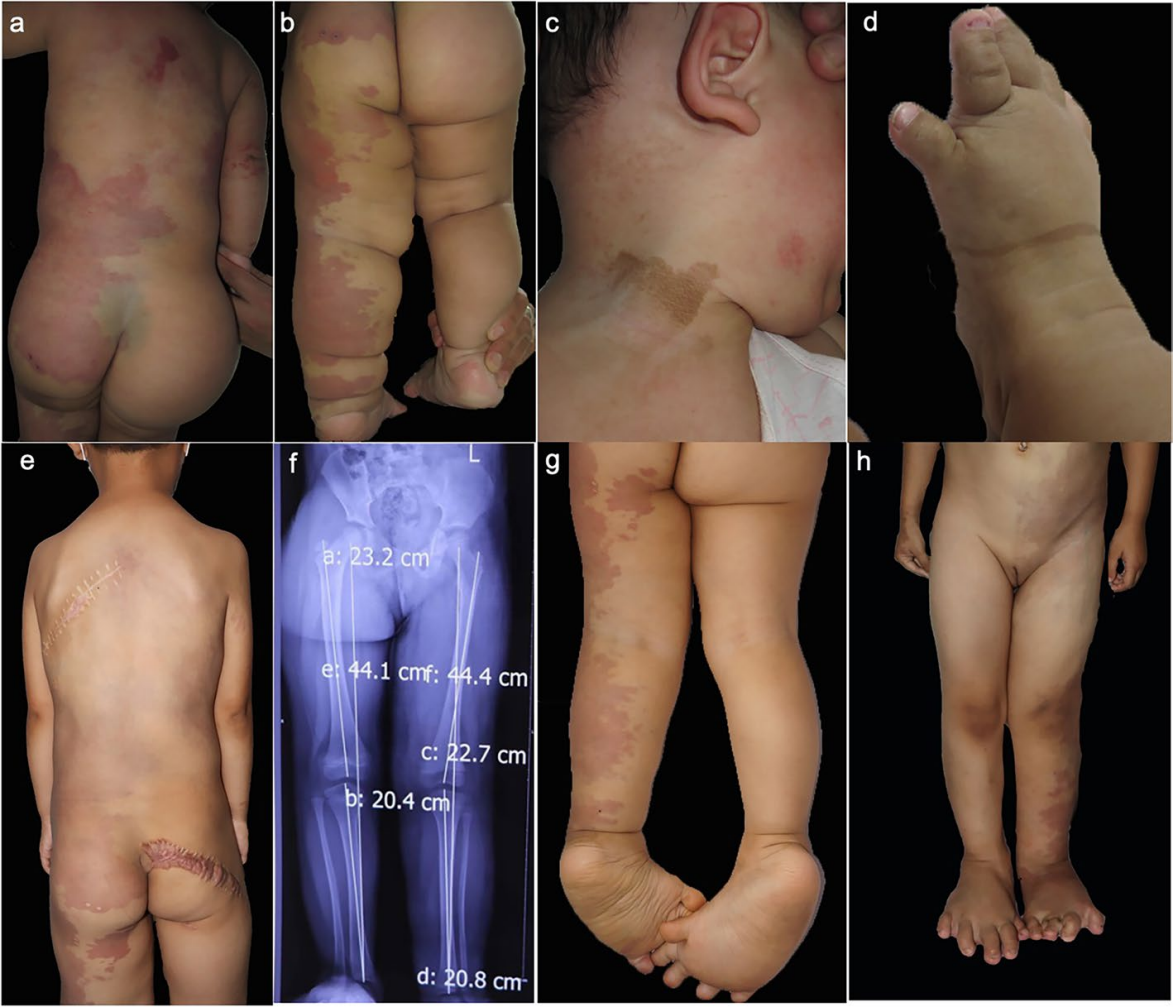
Clinical features of the patient. a-b The patient showed irregularly extended capillary malformations and asymmetrical hypertrophy of the lower limb. c Epidermal nevi. d Sandal gap toe and wide foot with second and third toes macrodactyly caused by skeletal elongation and adipose overgrowth. e Scoliosis and surgical removal of lipomatous masses. f X-ray examinations confirmed the asymmetrical overgrowth. g Decreased vascular anomalies and fat overgrowth of her lower limb after oral rapamycin treatment. h Removal of the left third toe by surgical amputation
